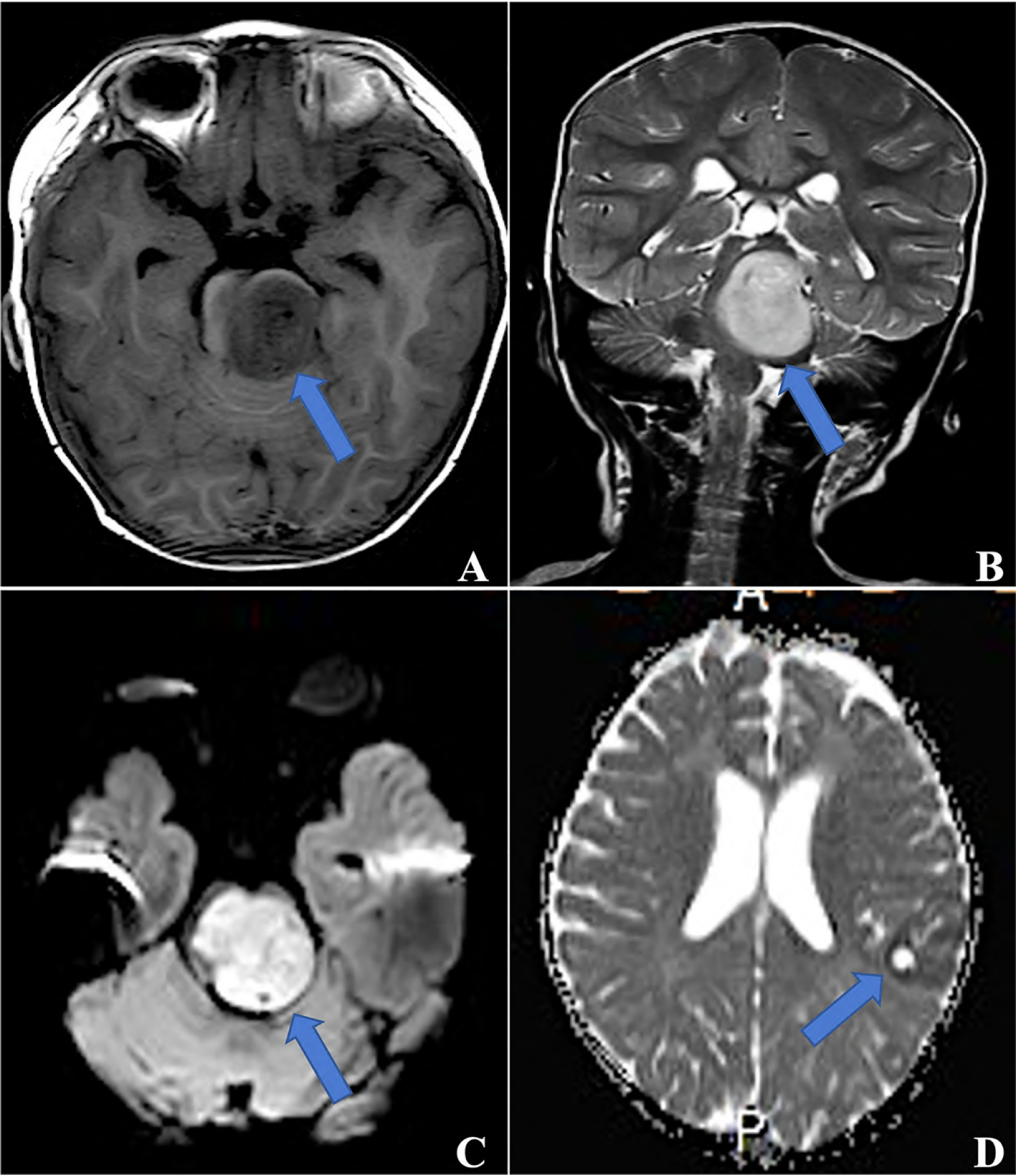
MRI for ETMRs, (A) TIWI showed a hypointense mass in the brainstem, which was hyperintense on both T2WI (B) and DWI (C) (blue arrows, case 5); (D) a 3×2×1 mass in the left parietal and occipital lobes with ringlike contrast enhancement (blue arrow, case 6).
